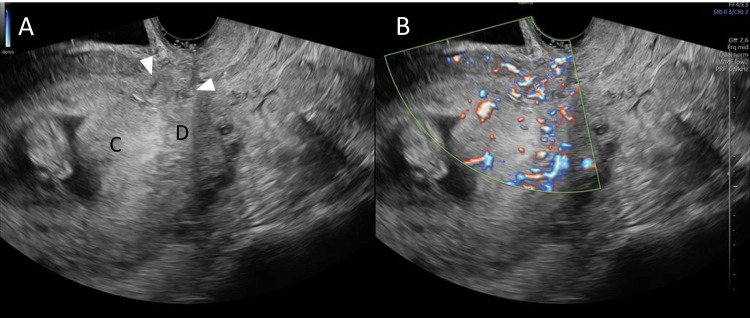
Sagittal transvaginal ultrasound B-mode imaging (A) and color Doppler imaging (B) at 12 weeks gestation (A) Similar to the MRI image, the lower myometrium is deficient, and the area is observed to have penetrating decidual tissue toward the anterior vaginal fornix (arrowhead). The boundary between the decidual tissue (D) and the chorionic villous tissue (C) can also be confirmed as in the MRI image, and by comparing the MRI image and the ultrasound image, a more detailed evaluation of the tissue characteristics can be performed. (B) When the same region is observed using color Doppler imaging, the decidual and chorionic villous tissue exhibit different blood flow patterns, making the boundary between them more distinct. MRI: magnetic resonance imaging
